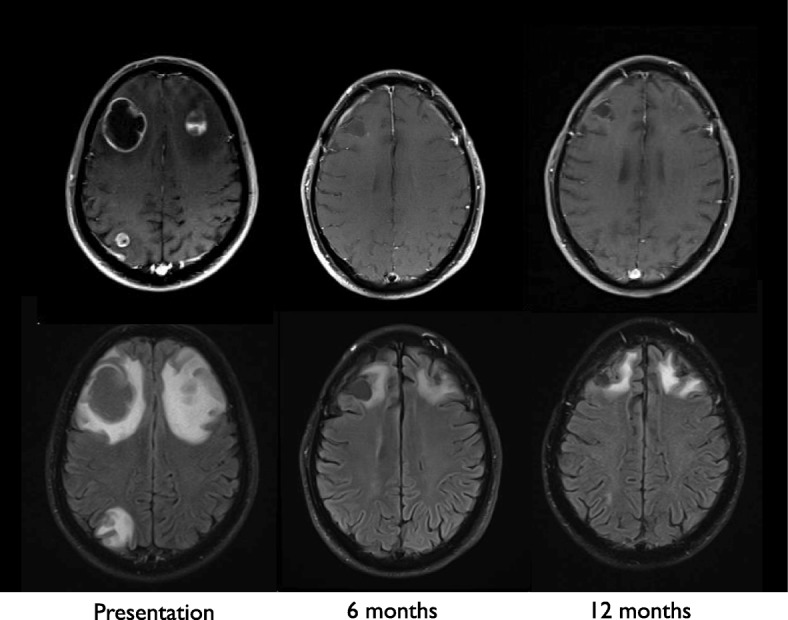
Magnetic Resonance Imaging (MRI) brain: T1-weighted images following intravenous gadolinium-based contrast (top panel) and axial FLAIR images without contrast (bottom panel). MRI brain images taken at initial presentation show two large frontal lobe enhancing lesions at the gray-white matter junction with significant surrounding edema and associated T2 FLAIR hyperintensity. Post-treatment changes remain evident at 6 and 12 month follow-up scans
